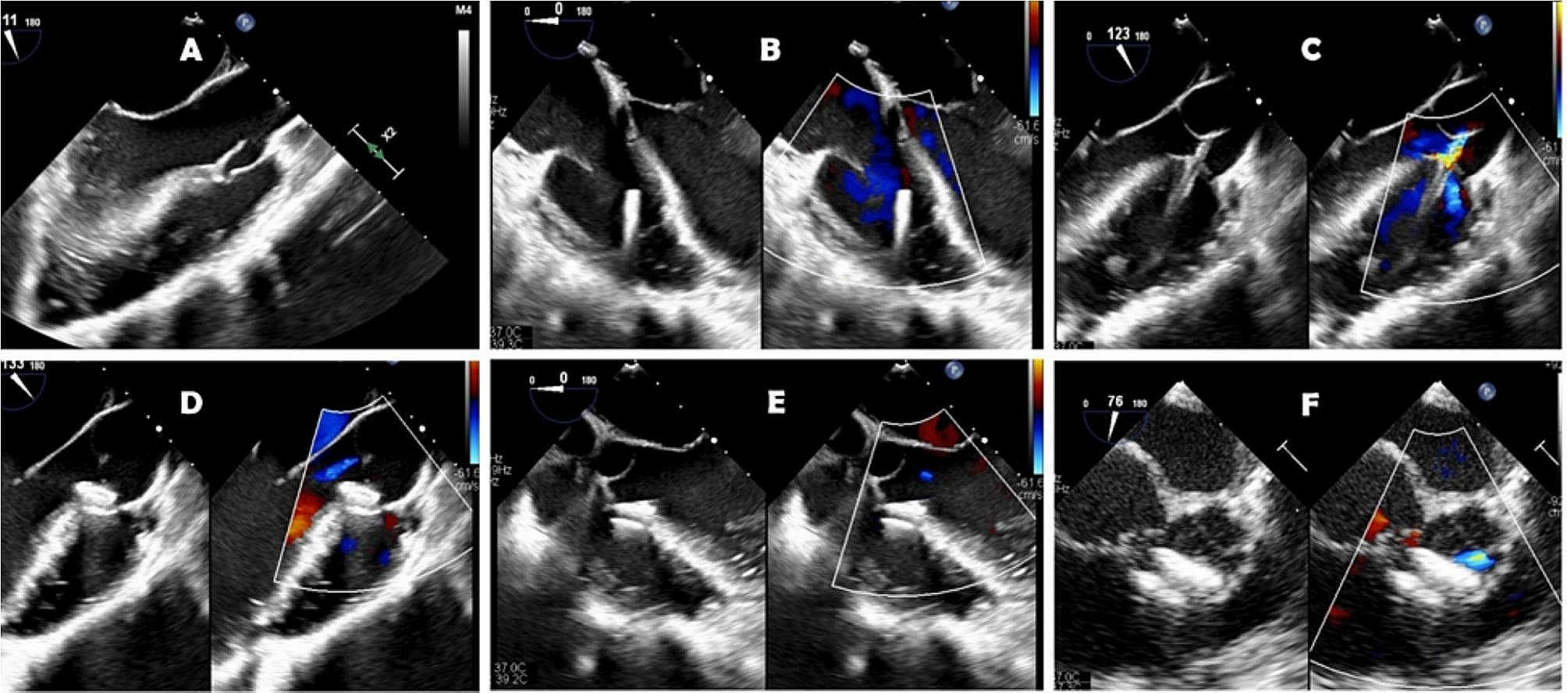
Serial transesophageal echocardiography images obtained during device implantation: A- prolapse of aortic valve in one patient, B- guide wire in the right ventricle, C- catheter in the left ventricular outflow tract, D- VSD device in the sagittal TEE view (12–130), E- device in five chamber view TEE angle 0, F- VSD device in short axis view
